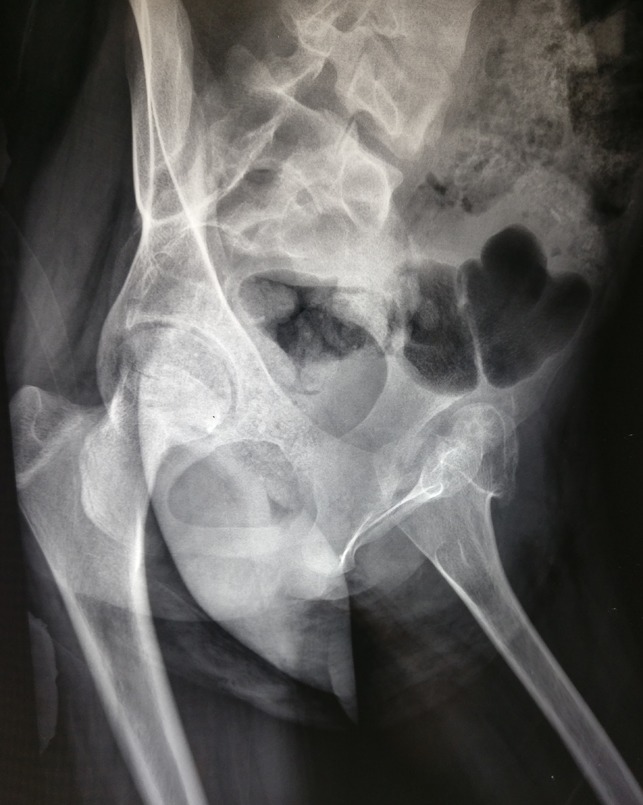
Attempted lateral radiograph demonstrating a deformed femoral head and pseudoacetabulum articulation.
